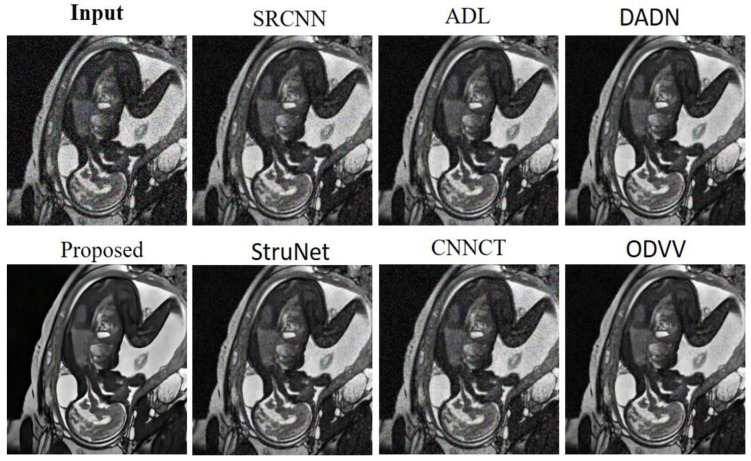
Outcomes from the denoising models tested on the Heart MRI dataset.
